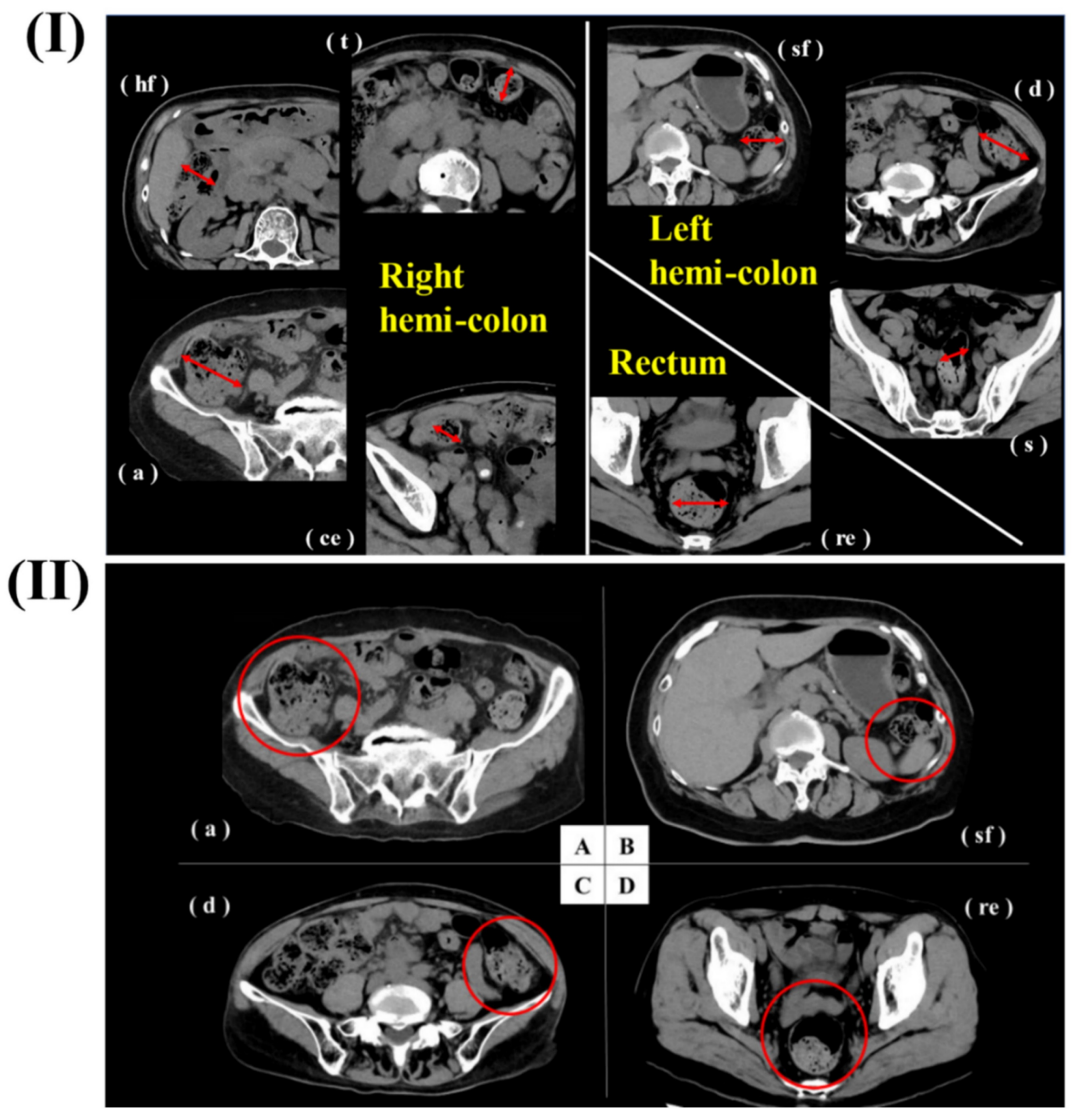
(I) A straightforward CT scan of the abdomen and pelvis has been obtained at each location of the right hemicolon, left hemicolon, and rectum. In the most dilated part of the intestine, which is represented by the red line, the diameter was measured (II) Representative cases. a) The stool volume was rated as four, and the gas volume was rated as two (shown by the red circle) using the CT scan. B) According to the CT picture, the volume of the stool was rated as 1, while the volume of the gas was rated as 2 (red circle). C) The stool volume was rated as three, and the gas volume was rated as two (shown by the red circle) using the CT scan. d) Based on the CT picture, the stool volume was rated as three, and the gas volume was also rated three (the red circle). Reproduced with the permission from ref 128. Fig.1, and Fig.2. (MDPI©2023).
